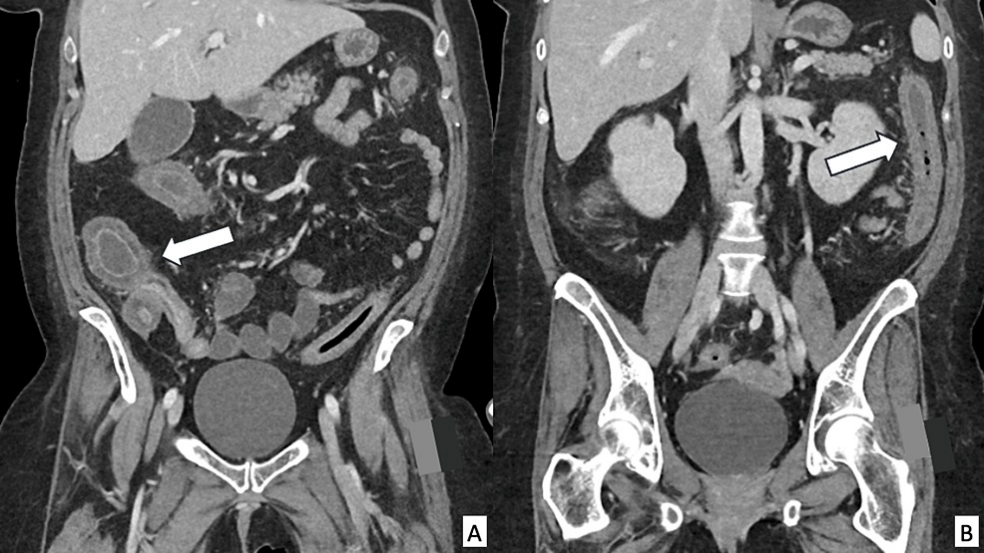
CT of the abdomen five months following admission Significant pan colonic mural thickening, including the cecum, terminal ileum (A), and descending colon (B)
